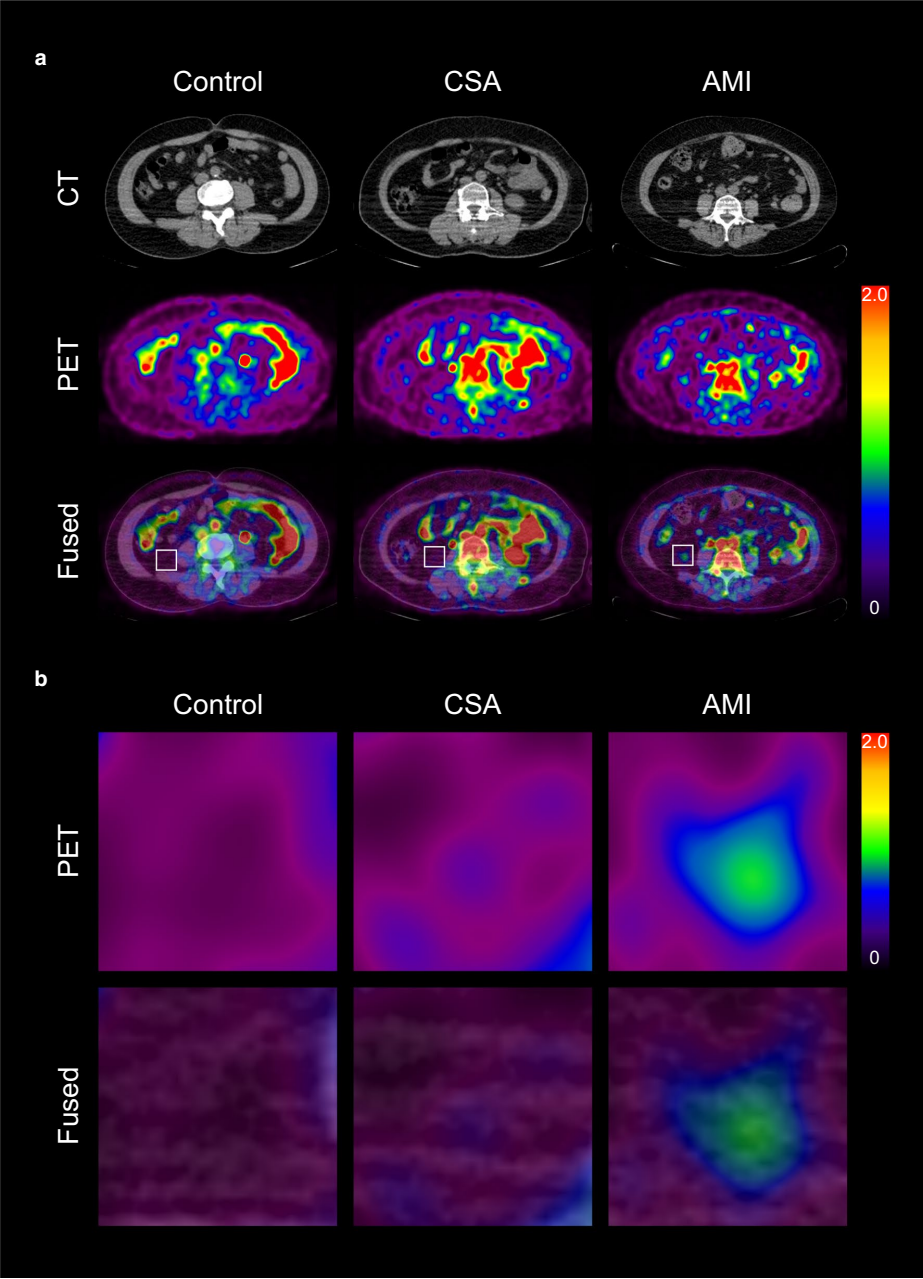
Representative images of visceral adipose tissue (VAT) glucose uptake according to the severity of coronary artery disease (CAD) (a), and their corresponding magnified views (b). *CSA* chronic stable angina, *AMI* acute myocardial infarction, *CT* computed tomography, *PET* positron emission tomography
